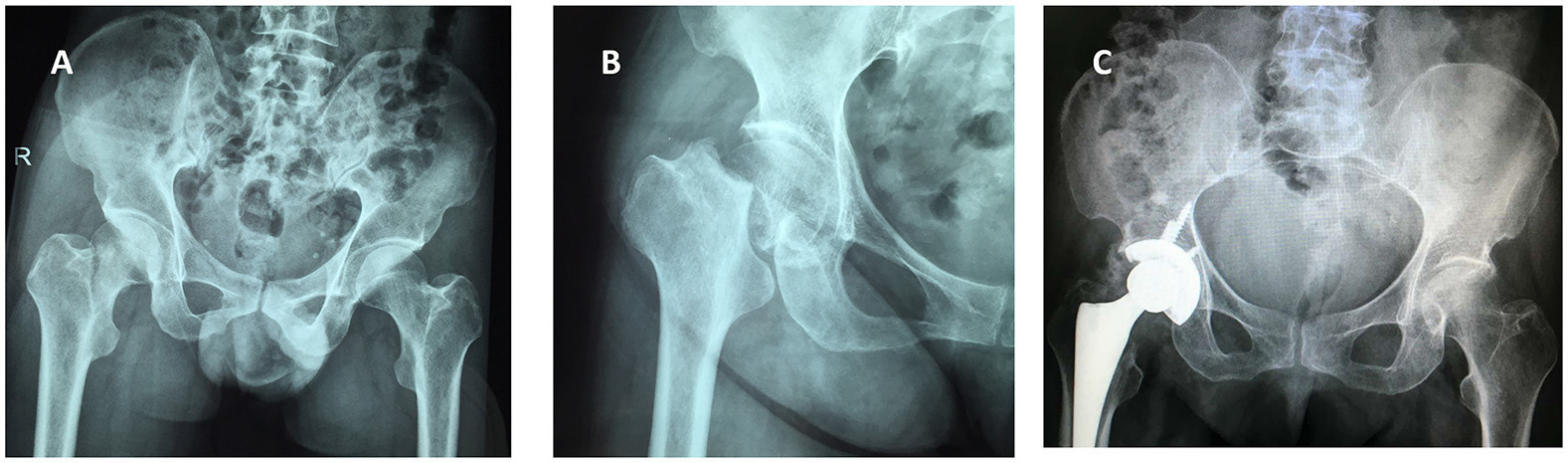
X-ray images of the right femoral neck fracture in a 65-year-old male patient with chronic renal failure before and after total hip arthroplasty. (A) Preoperative imaging shows compression fractures on the femoral neck of the right hip. (B) Representative images of the right femoral head fracture in lateral projection. (C) Representative images taken 3 days after total hip arthroplasty.
